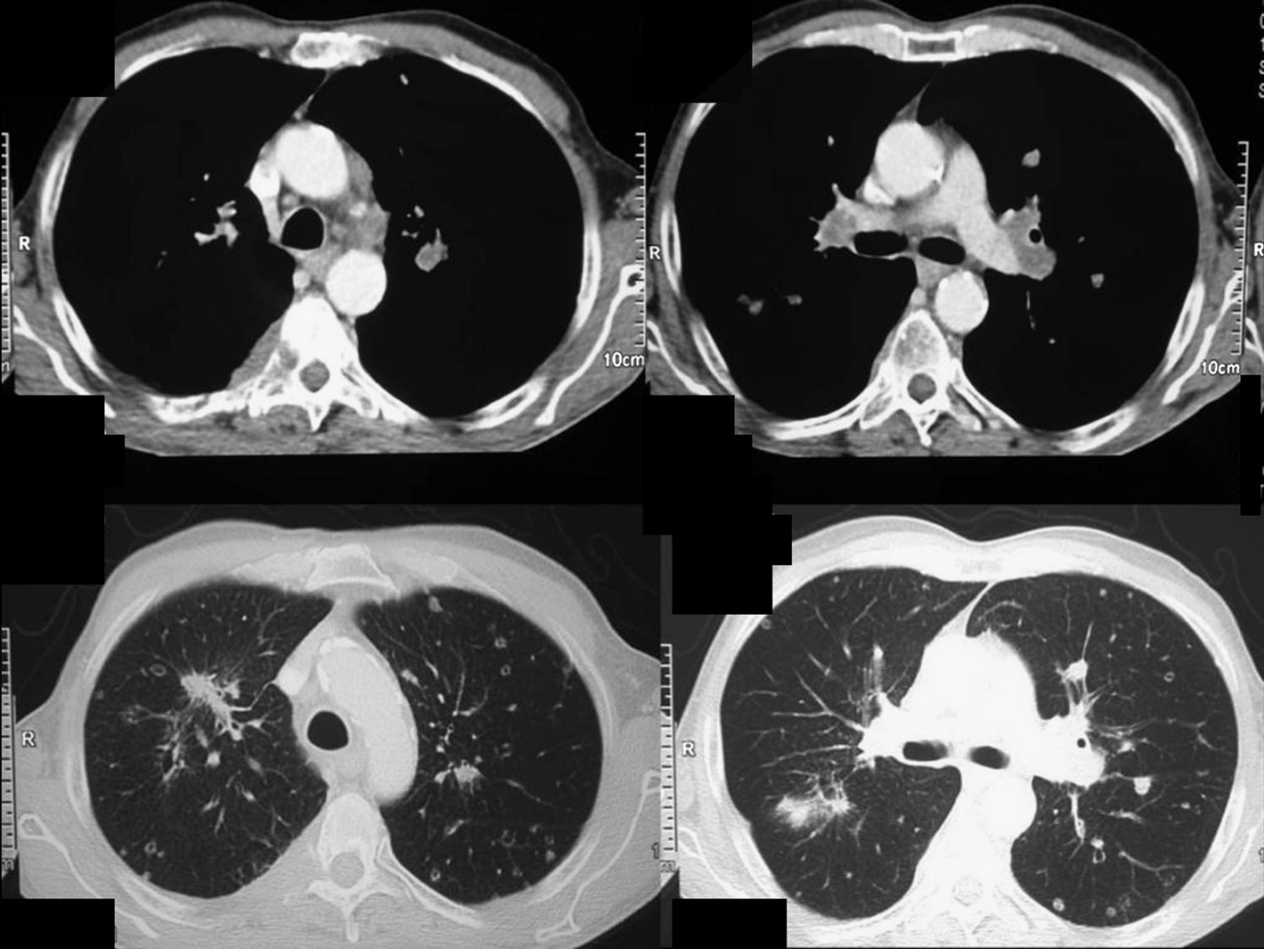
Computed tomography images of the thorax. (a) Enlarged lymph nodes in the aortopulmonary window. (b) Enlarged hilar nodes. (c) Spiculated mass in the apical segment of the right upper lobe and metastatic foci. (d) Metastatic pulmonary nodules.
